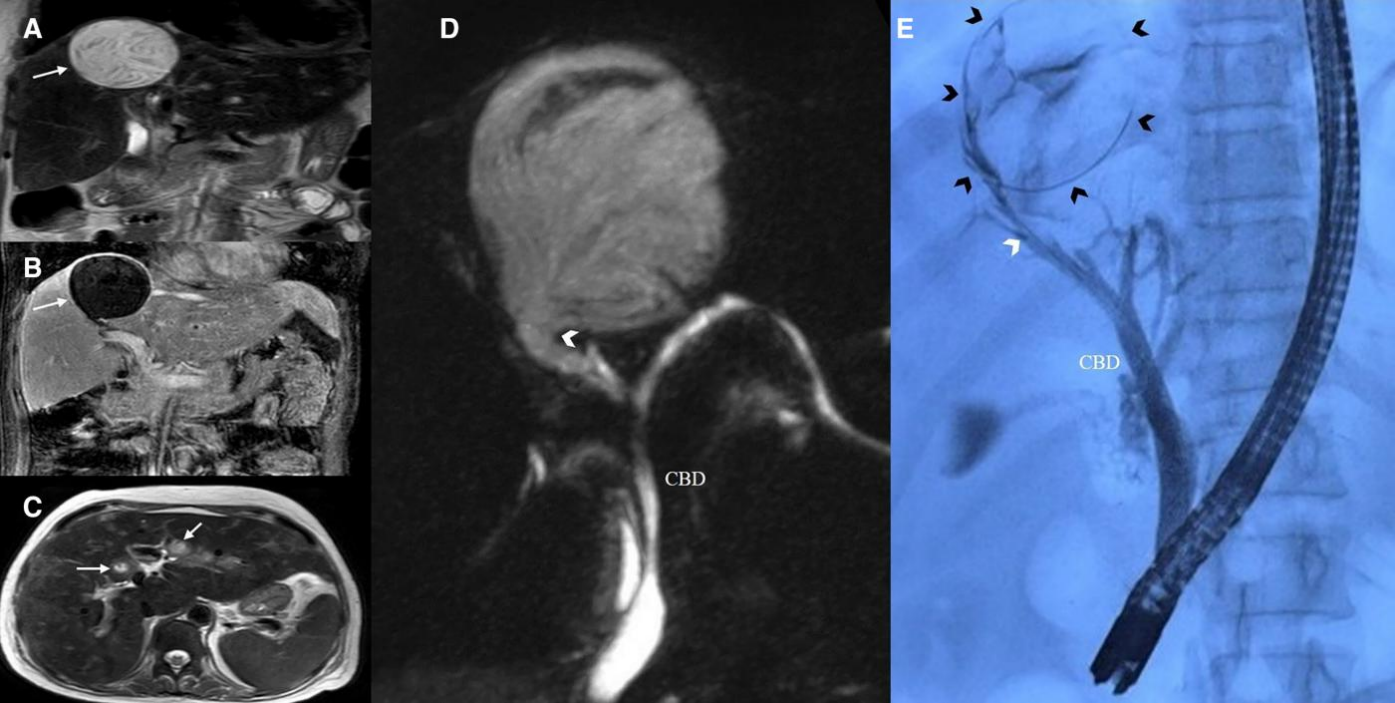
(A–C) MRI showed a well-defined, rounded, hyperintense lesion with thin internal septations in segment VIII, suggestive of a hepatic hydatid cyst. (D) Magnetic resonance cholangiopancreatography revealed communication between the segment VIII lesion and right hepatic duct. (E) Cholangiography revealed extravasation of contrast medium into the cyst, confirming cystobiliary communication.
